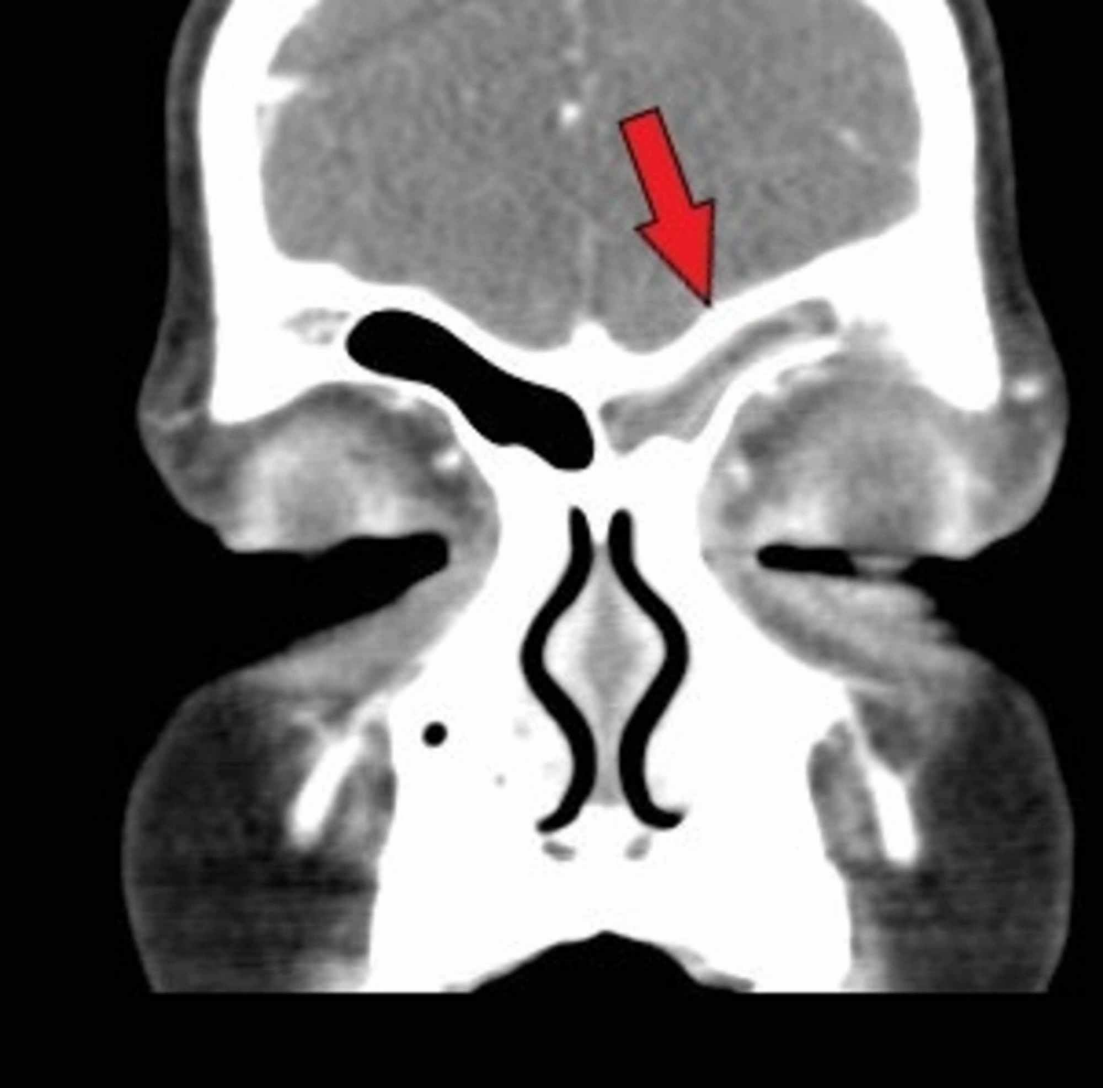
Coronal CT scan with arrow indicating opacification of the left frontal sinus
